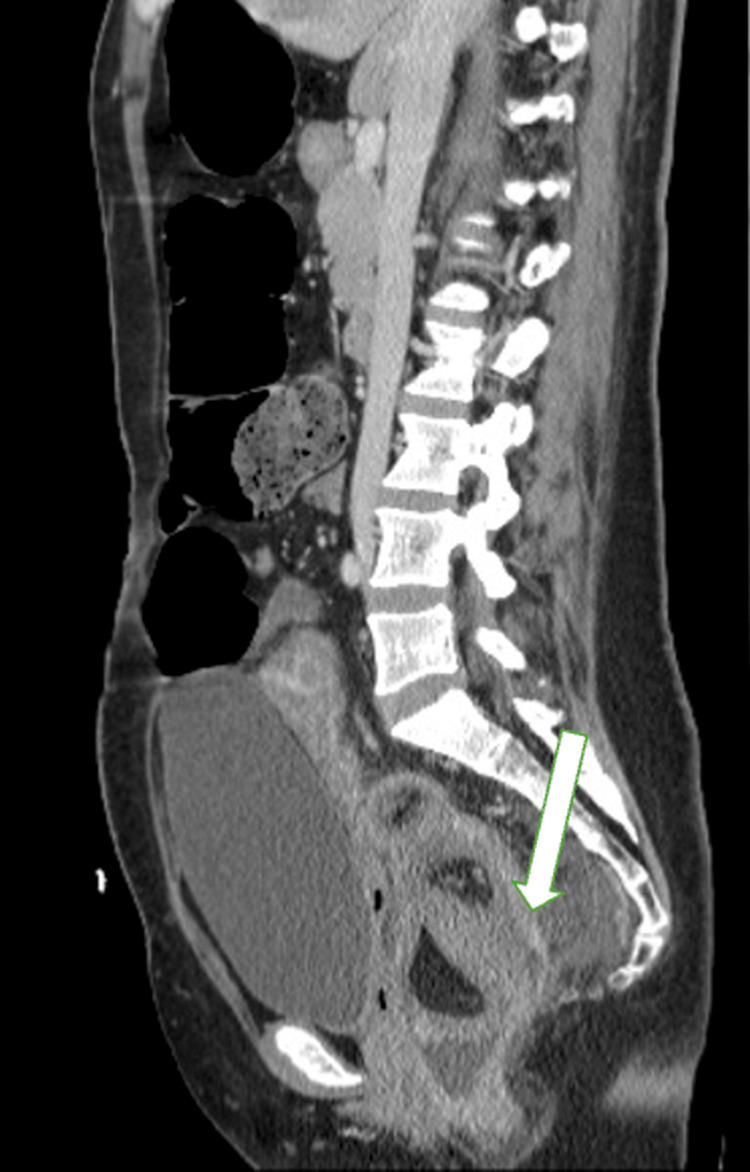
CT abdomen and pelvis, sagittal view, demonstrating rectosigmoid intussusception (arrow).
